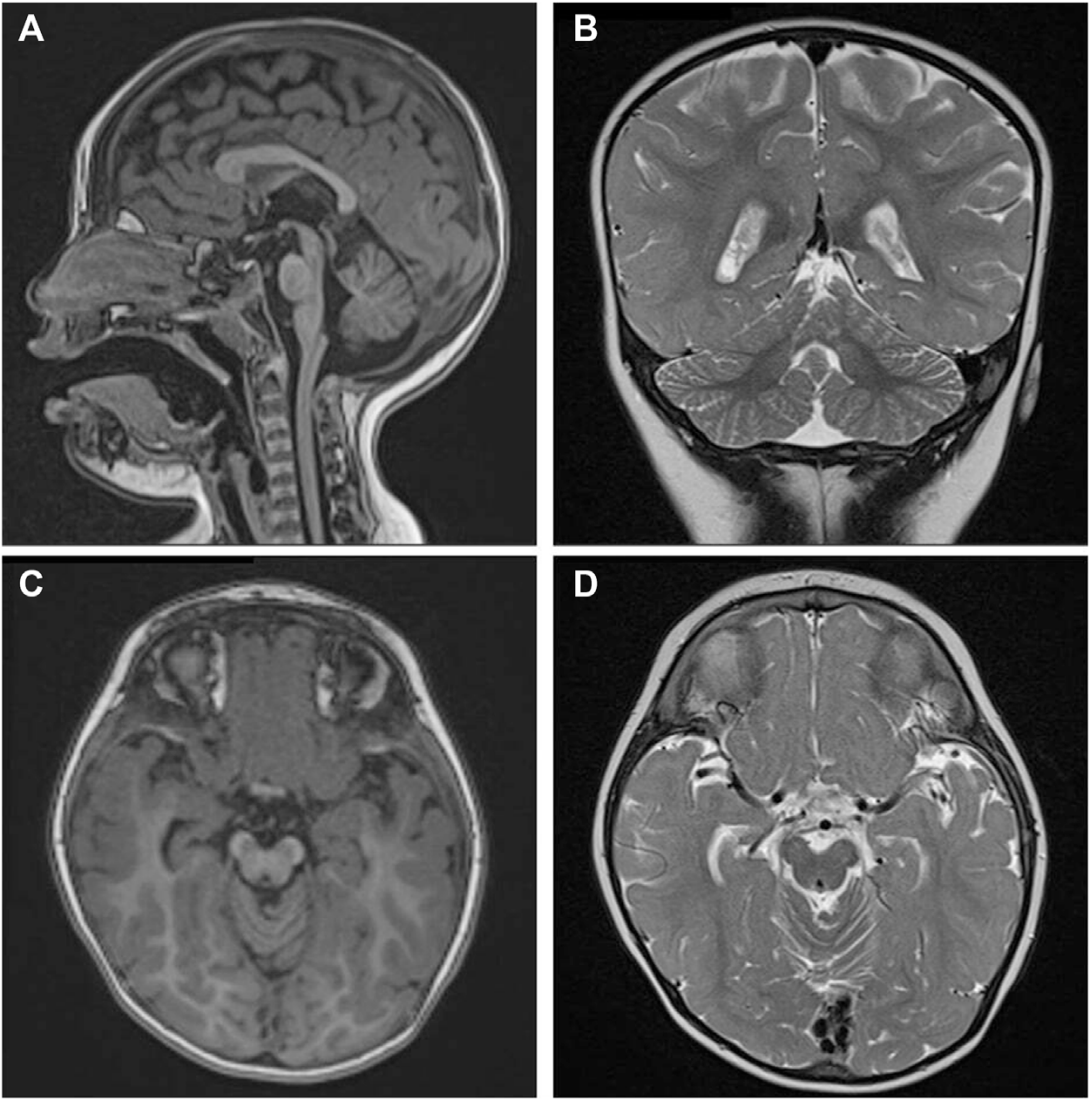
Brain Magnetic Resonance imaging (MRI) analyses of the patient. (A) Mid sagittal T1-weighted image and (B) Coronal T2-weighted image demonstrating cerebellar vermis hypoplasia (B). (C) Axial T1-weighted and (D) T2-weighted images showing mild asymmetry of cerebral peduncles.
